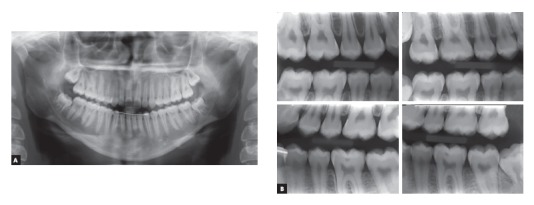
Final panoramic (A) and interproximal (B) radiographs.
